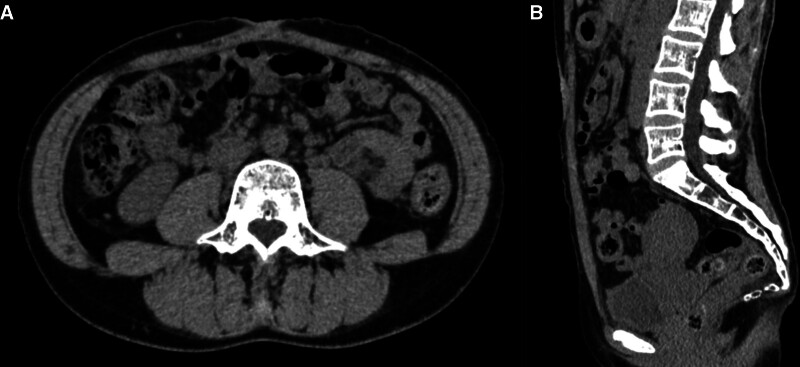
Computed tomography images 1 month after the delivery. Axial (A) and sagittal (B) views showing no recurrence of diastasis recti abdominis and no seroma.
